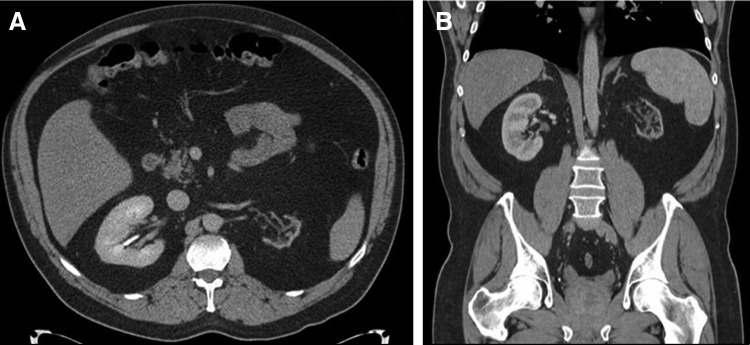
Axial (A) and coronal (B) CT slices demonstrating an atrophic *left* kidney without any identifiable mass or lesion.
